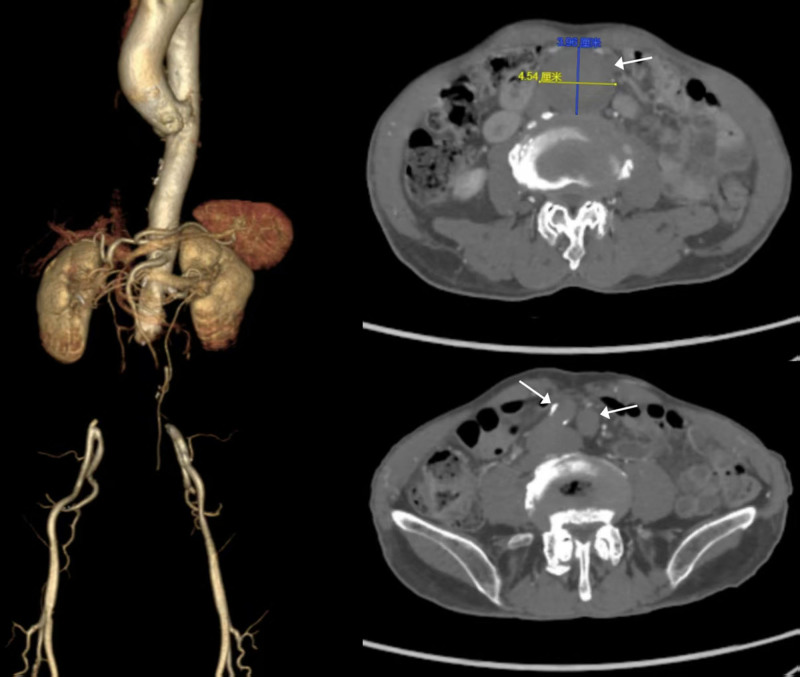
Preoperative CTA showed acute thrombosis of a 4.54 cm infrarenal AAA and both common iliac arteries (white arrows). The proximal neck of the AAA was patent and suitable for EVAR. AAA = abdominal aortic aneurysm, CTA = computed tomography angiography, EVAR = endovascular aneurysm repair.
